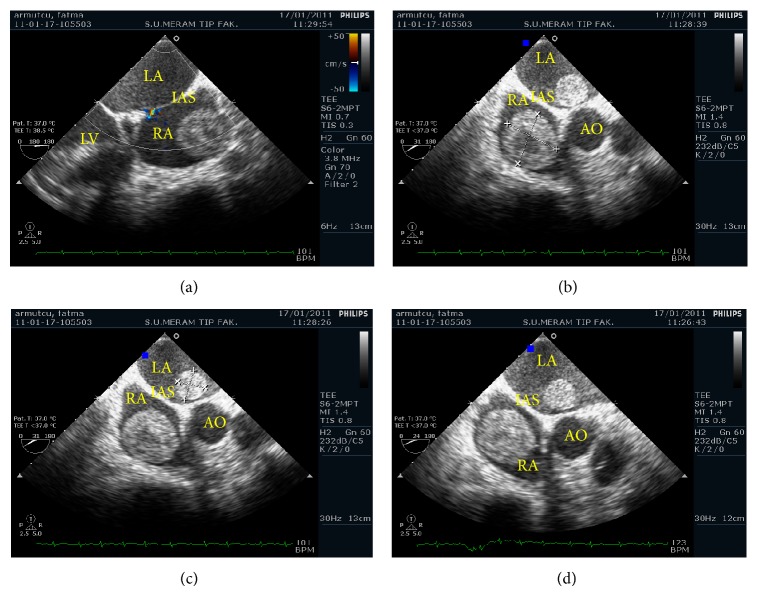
Transesophageal echocardiography demonstrates two mobile masses with biatrial cardiac metastases of malignant melanomas in uterine cervix, one attached to free wall (29 × 21 mm) in right atrium and the other attached to interatrial septum (20 × 17 mm) in the left.
